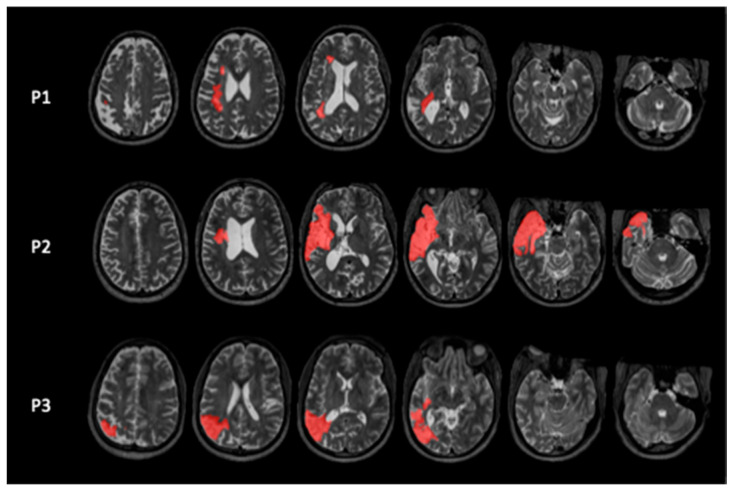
T2-weighted MRI scans showing the location of left hemispheric lesions in red.
